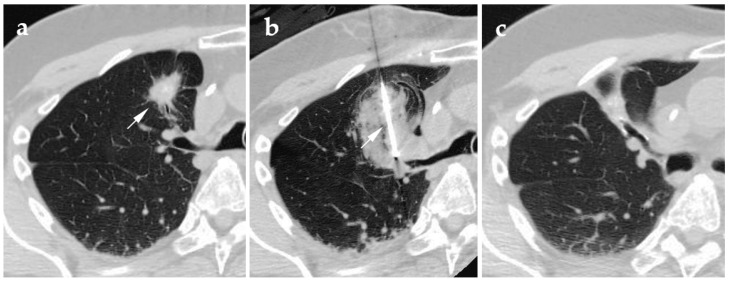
58-year-old male presenting with colorectal metastases to the lung, biopsy proven post-radiation therapy recurrence. (a) Supine axial pre-ablation CT with the white arrow indicating the lesion to be ablated; (b) supine axial CT of the probe (white arrow) placement during the tumor cryoablation; (c) supine axial follow-up CT at 2 years post-ablation.
